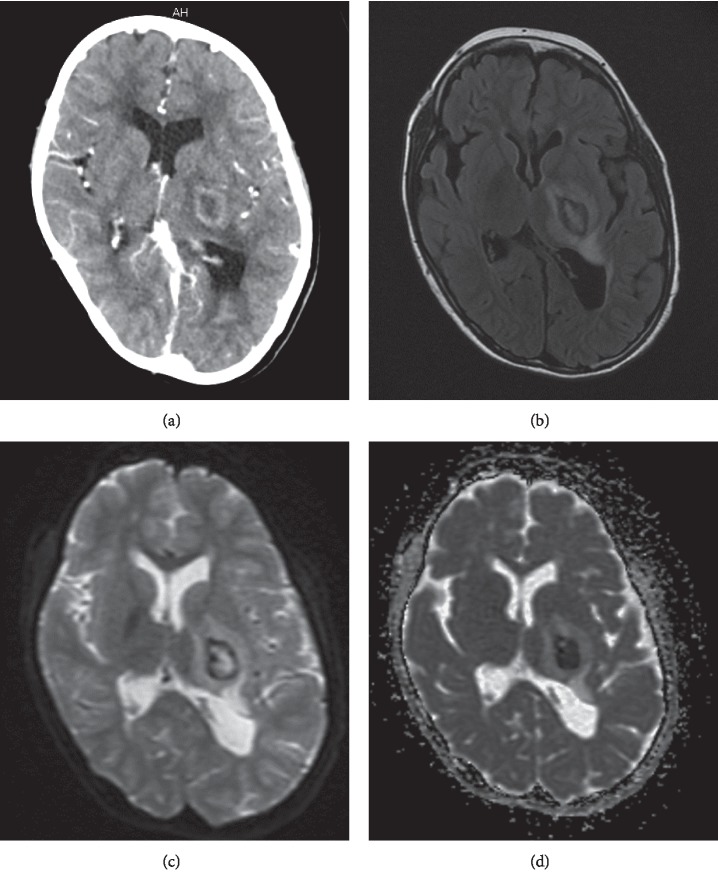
Brain abscess detected by contrast-enhanced computed tomography and magnetic resonance imaging. (a) Contrast-enhanced computed tomography scan showing a low-density area with ring enhancement. (b) T2-weighted fluid-attenuated inversion recovery image showing a hyperintense lesion surrounded by a thin hypointense ring. Hyperintense edema is noted. (c) Diffusion-weighted magnetic resonance image demonstrating a hyperintense signal within the lesion. (d) Apparent diffusion coefficient map shows hypo-intensity within the lesion, confirming the presence of restricted diffusion.
